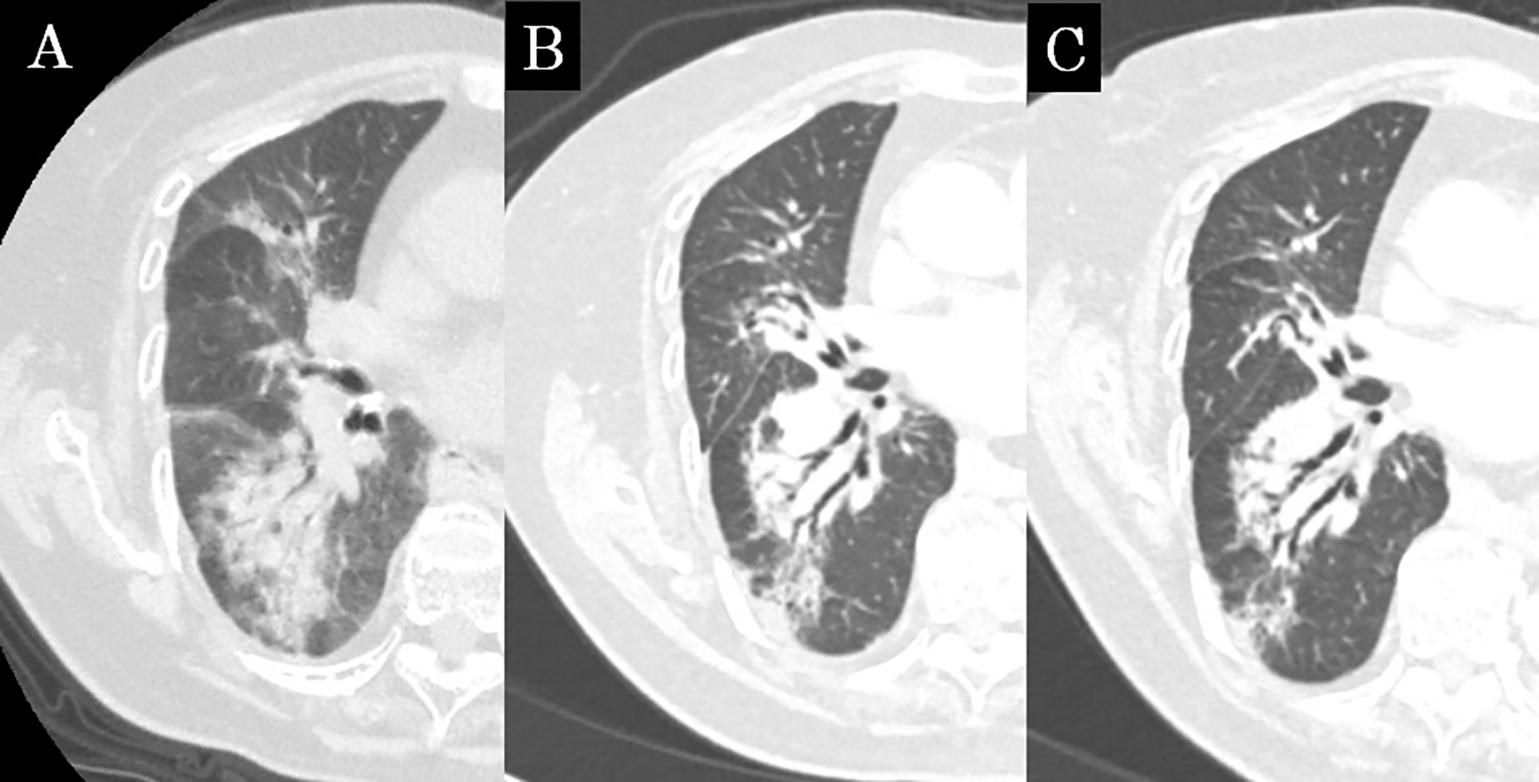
Concordance CT appearance, case 1. (a) The shadow extends to the right middle and lower lobes beyond the high-dose region; this was diagnosed as diffuse consolidation at 6 months after SBRT. (b) The shadow shows consolidation, volume loss and bronchiectasis; this was diagnosed as the modified conventional pattern at 21 months after SBRT. (c) The shadow remained at 45 months after SBRT.
